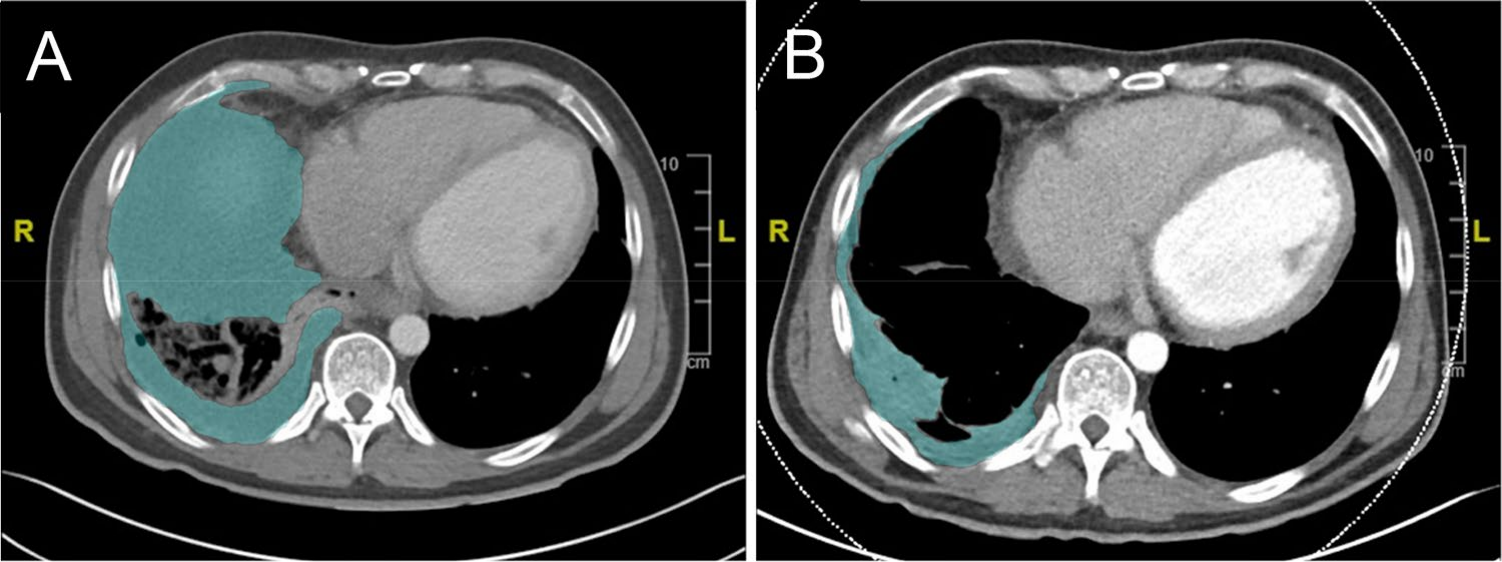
Pre- and post-treatment CT-volumetry of the empyema cavity. Exemplary transverse plane CT imaging with the borders of the empyema cavity shaded in green: pre- (A) and post-treatment (B).
